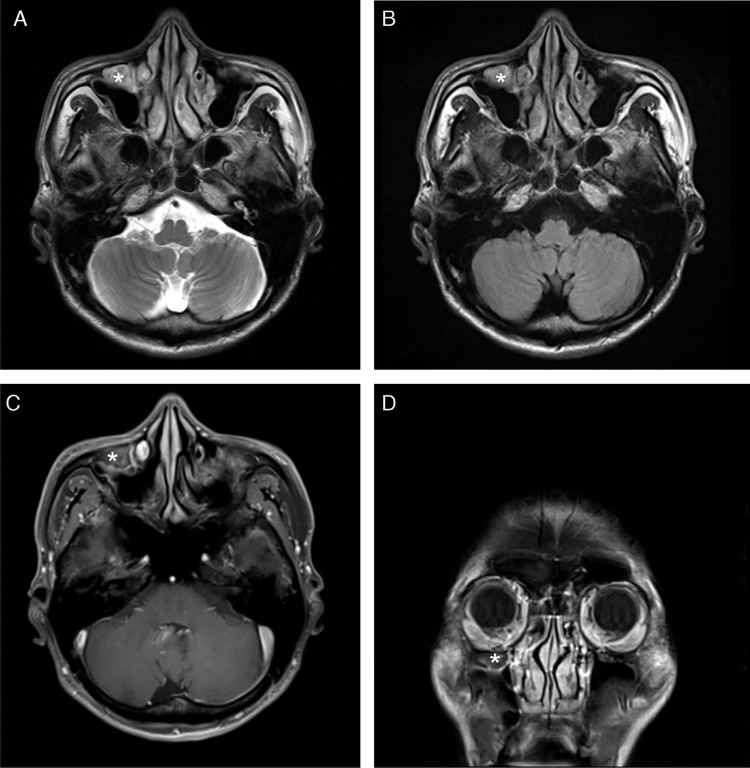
Brain MRI showed a fluid-filled Haller's cell (*) in the roof of the right maxillary sinus that was hyperintense on T2 axial image (A) and T2 FLAIR image (B). It was isointense on T1-weighted axial image (C) with subtle postcontrast enhancement (D).
